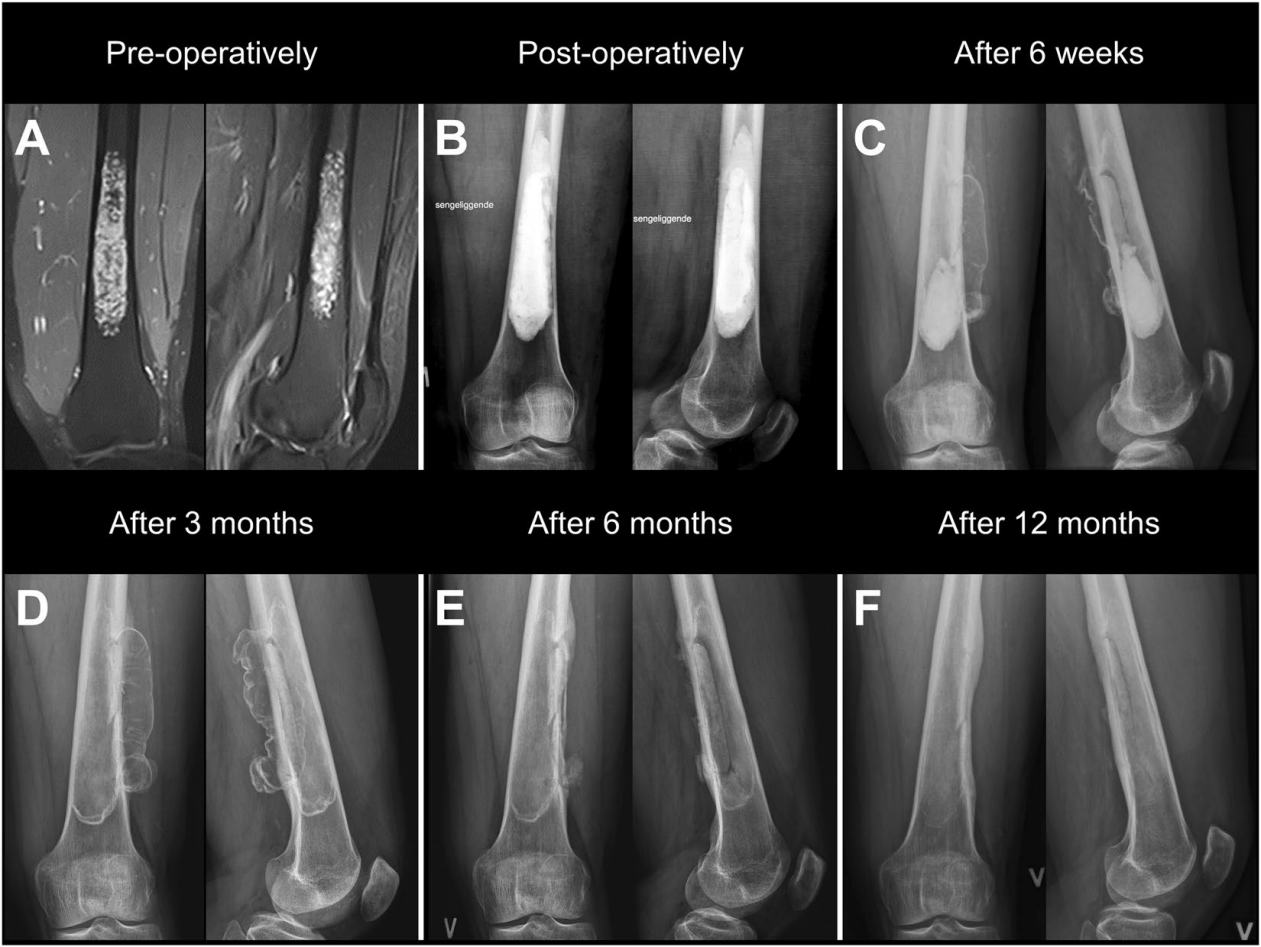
A patient treated by curettage and defect filling, using a combination of CERAMENT|G (20mL) and CERAMENT|BONE VOID FILLER (36mL) via an extended cortical window, for a large enchondroma in the left distal femur. (A) Pre-operative MRI-scan. (B) Post-operative x-ray without product-leakage (C,D) X-rays after 6 weeks and 12 weeks showing soft-tissue leakage. (E) X-ray after 6 months showing almost complete resorption of soft-tissue leakage. Outline of the bone window is clearly visible. (F) X-ray after 12 months showing complete resorption of the soft-tissue leakage, blurring of the bone window, and thickening of the posterior and lateral cortex.
